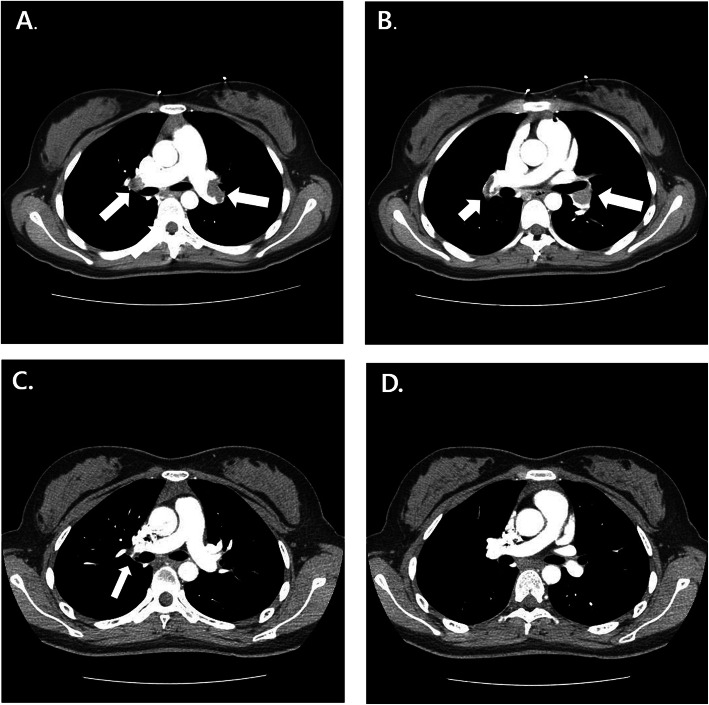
Chest computed tomography (CT) scan with contrast showing multifocal thromboemboli (White Arrow) in both pulmonary arteries (a and b). Repeated CT scan performed six months after anticoagulation therapy showing complete resolution of the thromboemboli of the main branches of both pulmonary arteries (c and d)
